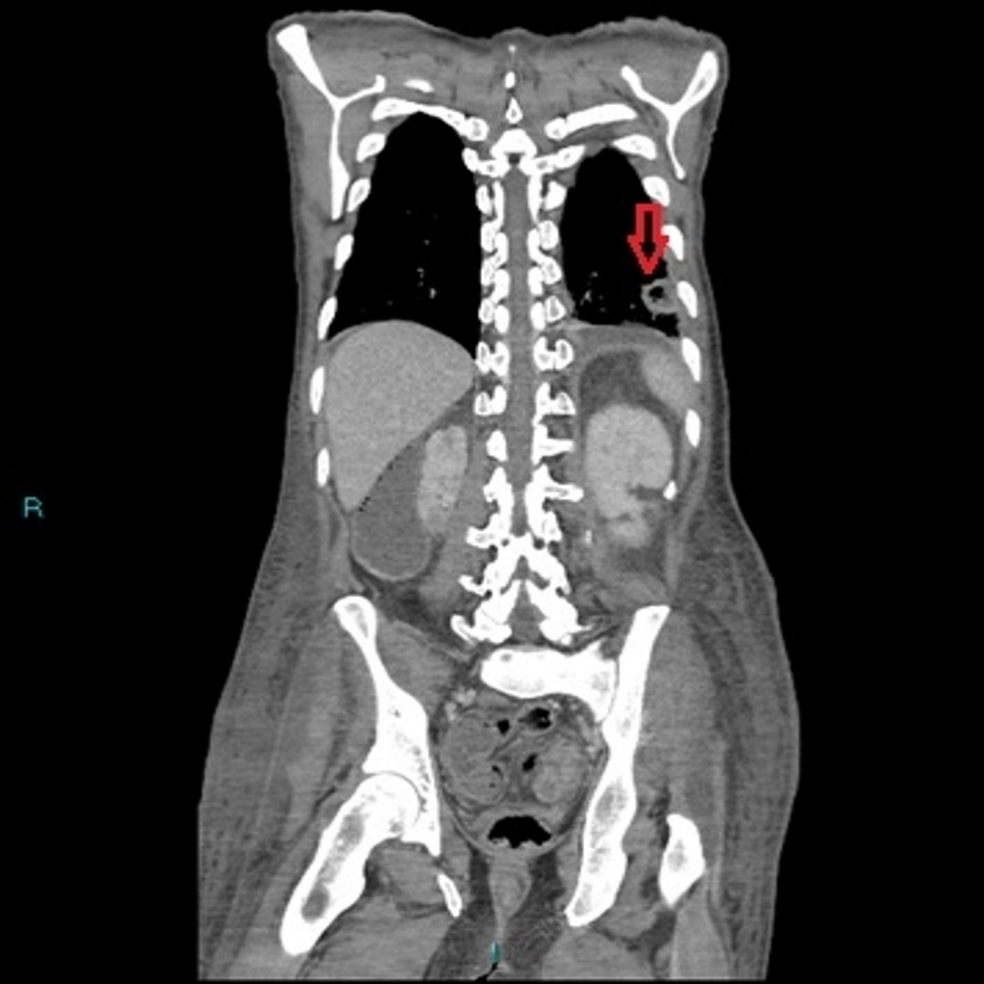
CT thorax, abdomen, and pelvis (TAP) showing a 2.5 cm, sub-pleural, wedge-shaped lesion over the lateral basal segment of the left lower lobe with adjacent atelectasis and small pleural effusion suggestive of abscess
